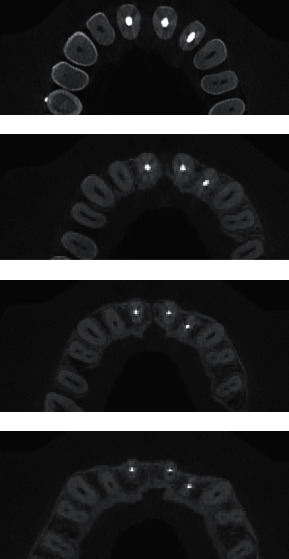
CBCT images—3 years after replantation.
